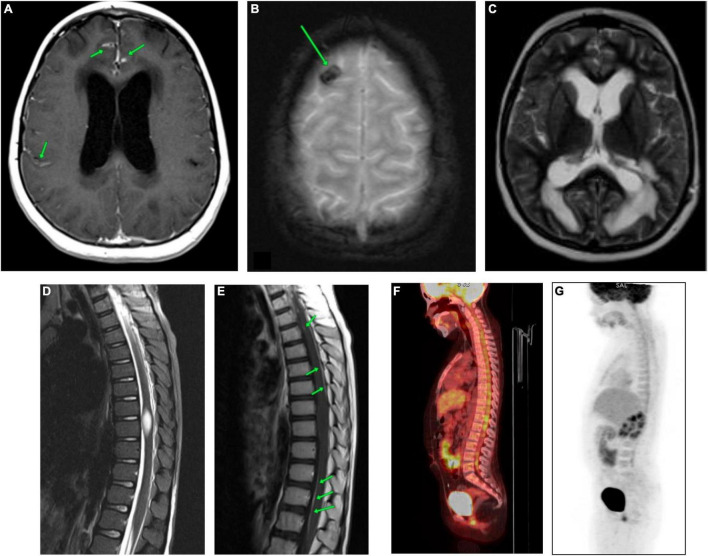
CNS MRI and the whole-body PET-CT of the patient carried out at the time of admission to the Almazov National Medical Research Centre. (A) Brain MRI (CE T1-WI). Diffuse infiltration of the meninges (soft and hard) of the cerebral hemispheres with uneven thickening and contrast accumulation. (B) Brain MRI (T2*-WI). The area of hemosiderin deposition in the cortex of the right frontal lobe. (C) Brain MRI (T2-WI). Triventricular hydrocephalus. Ventriculoperitoneal shunting (VPS), the shunt in the left lateral ventricle. Severe periventricular edema. Subarachnoid spaces are narrowed. (D) Spinal cord MRI (STIR). The spinal cord at the level of Th8-Th9 vertebrae is thickened, and a spindle-shaped formation of 22 mm x 9 mm x 8 mm is observed. (E) Spinal cord MRI (post-contrast T1-WI). Pia mater of the thoracic region of spinal cord evenly accumulates a contrast agent. The spindle-shaped formation shows no signs of contrast. (F,G) The whole-body [18F]2-fluoro-2-deoxy-D-glucose positron emission tomography/computed tomography (18FDG-PET-CT). The picture of diffuse heterogeneous hyperfixation of the radiopharmaceutical along the course of the cervical, thoracic, and lumbar segments of the spinal cord with a maximum value of standardized drug capture of 3.12.
